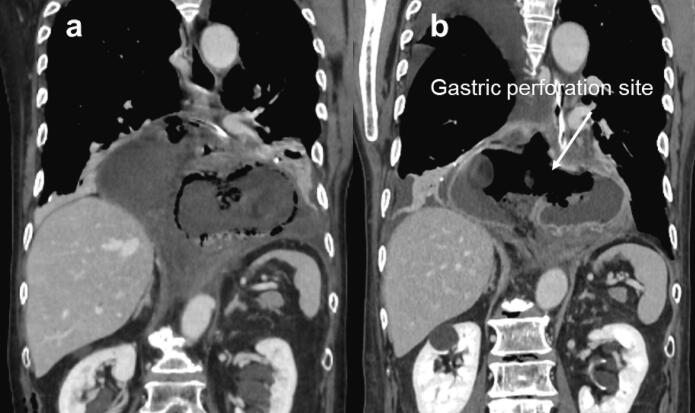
Computed tomography image showing (a) recurrence of the hiatal hernia and intramural surgical emphysema of the prolapsed stomach and (b) perforation of the gastric wall and free-air in the mediastinum.
